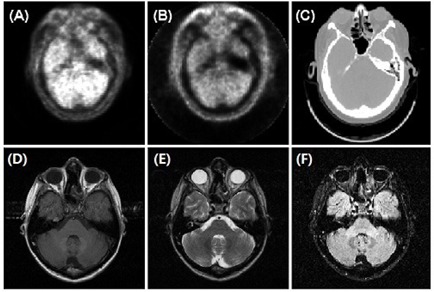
Two‐dimensional views of the common landmark (the skin) for PET with (a) and without (b) attenuation correction, CT (c) and MRI: T1 (d) T2 (e) and FLAIR (f).
